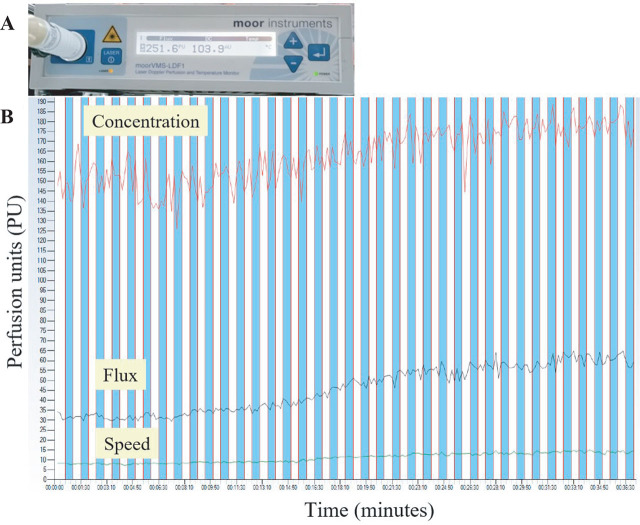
(A) High power LDF device and (B) data collection from HD patients. HD, hemodialysis; LDF, laser Doppler flowmetry.
